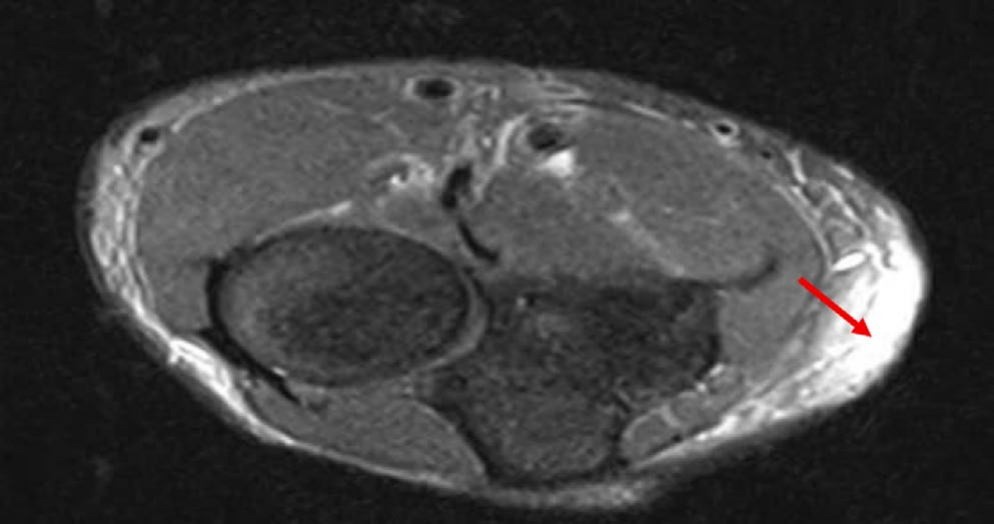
Elbow Magnetic Resonance Imaging (MRI). Edema in the subcutaneous tissue, especially on the medial side of the elbow, extending to the proximal forearm.
